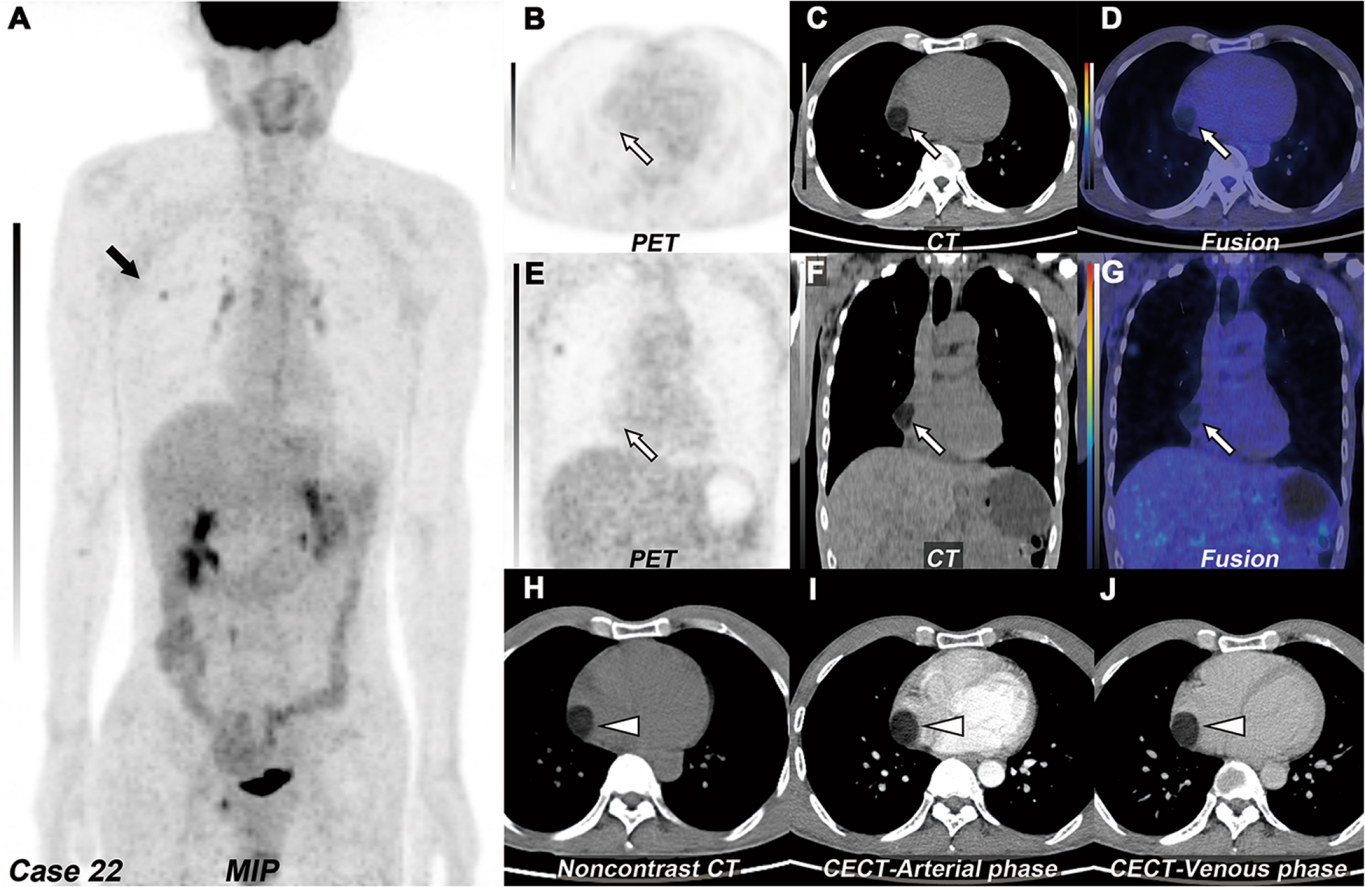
Right atrial lipoma. a MIP of ^18^F-FDG PET/CT revealed lung cancer with increased ^18^F-FDG uptake in the right upper lobe (black arrow). b–d The transverse and e–g coronal view of selected PET, CT, and fused PET/CT images revealed a well-circumscribed solitary mass, which located in the right atrium (white arrow, SUVmax 0.8). h On the non-contrast CT, the tumor presented as sharply margin, ovoid shape with fat density; the CT attenuation value was − 50 HU (white arrowhead). i–j The transverse view of arterial and venous phases of CECT images revealed no enhancement of the tumor
